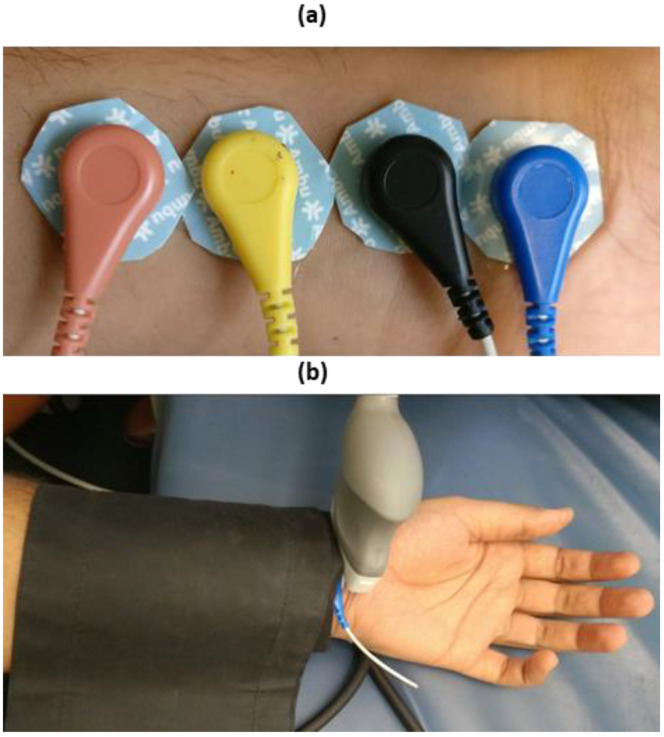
(a) Electrode setup for impedance measurements at forearm section. (b) Ultrasound and impedance measurement setup with cuff pressure at 200 mmHg.
